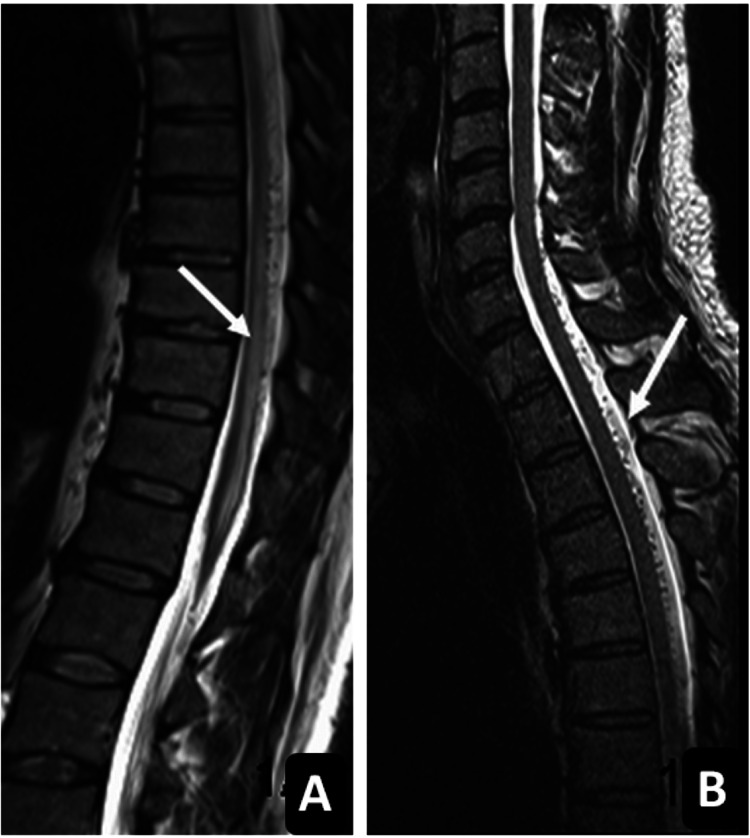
Sagittal T2-weighted MRI (A and B). In image A, a hyperintense intramedullary signal alteration from T7 to T12 represents edema (white arrow). In image B, the multiple flow voids in the dorsal subarachnoid space are distributed over almost the entire thoracic cord length, complying with pathological distended vessels (white arrow). MRI: magnetic resonance imaging
